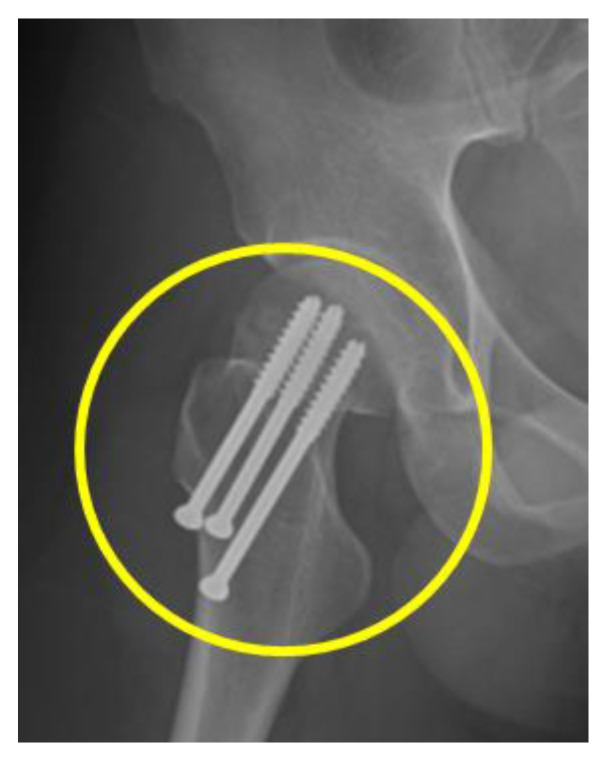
Lateral view X-ray of right hip femoral neck fracture, Garden type I post internal fixation of right femoral neck fracture with 6.5 mm cannulated screws × 3.
